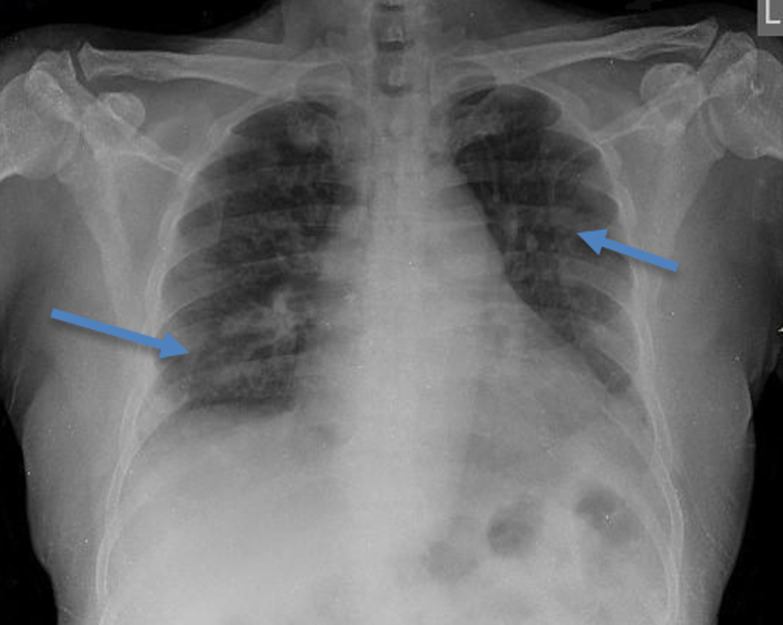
chest X-ray, bilateral parenchymal infiltrates likely of infective etiology
